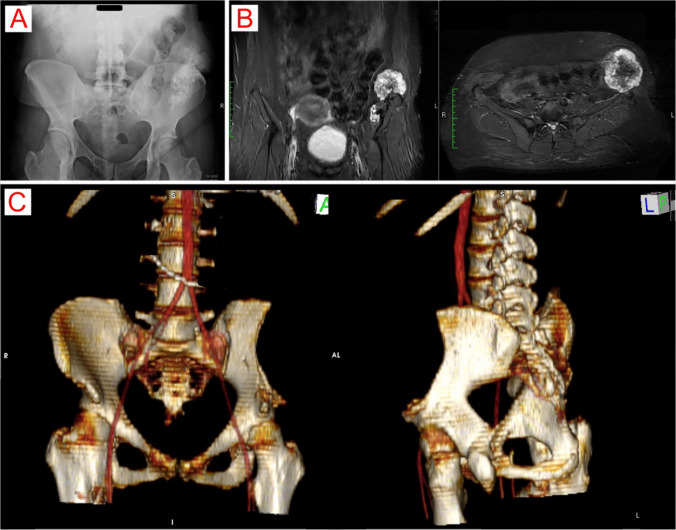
Thirty-eight-year-old female patient with Grade 2 CS of the left iliac wing arising from exostosis in the context of multiple hereditary exostosis; Preoperative X-ray (A) and STIR-MRI (B) showing the lesion; C) 3D reconstruction after removal of the lesion
